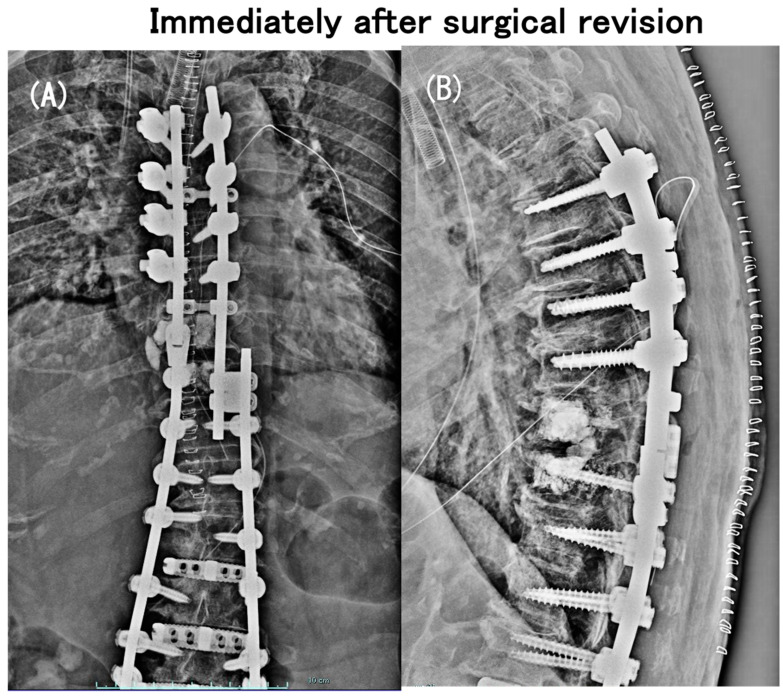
Coronal (A) and sagittal (B) full-length radiographs obtained at an early postoperative period of urgent surgical revision extending the posterior instrumented fusion proximally to T5 with spinal cord decompression.
